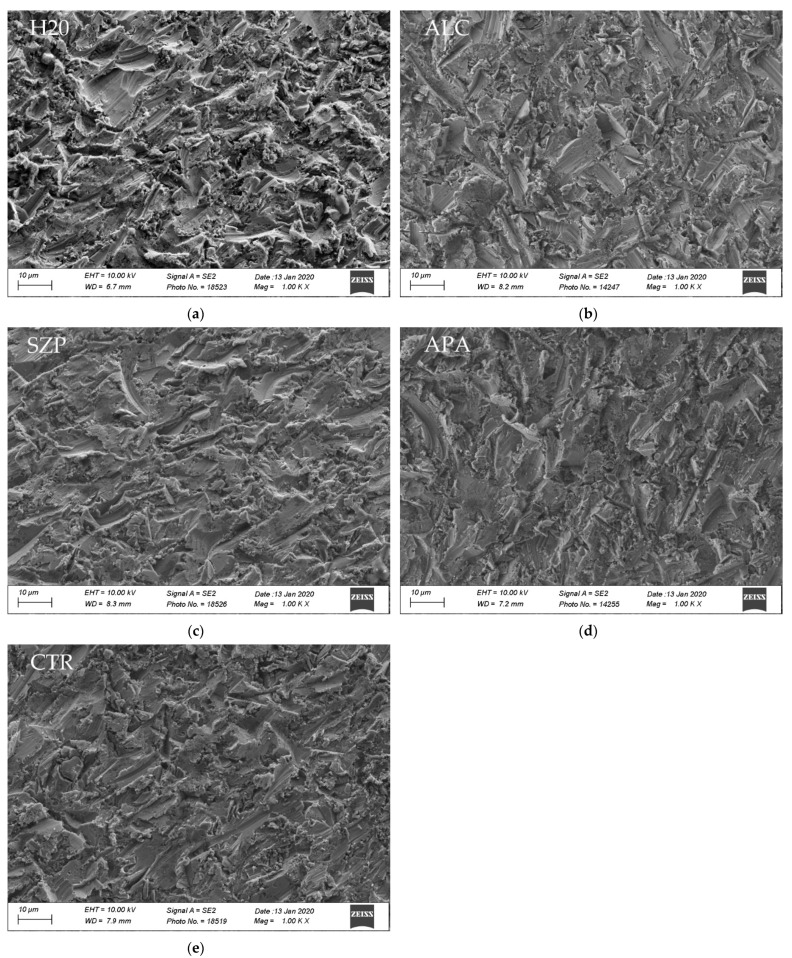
SEM photographs of the titanium bond surface of each group after cleaning (1000×): (a) water (H_2_O); (b) ultrasonic alcohol bath (ALC); (c) alkaline suspension of zirconium oxide particles (SZP); (d) reapplied standardized airborne-particle abrasion (APA); (e) control without contamination (CTR).
